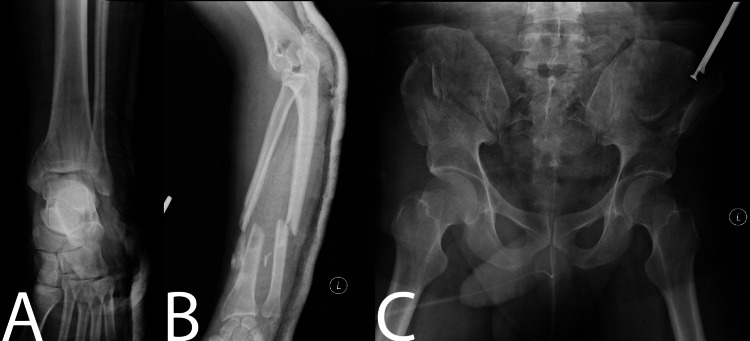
Skeletal survey radiographs. Radiographs showing (A) left medial malleolus fracture, (B) left forearm fracture, (C) bilateral iliac wing fractures as well as bilateral crescent fracture-dislocation extending to the sacroiliac joint.
